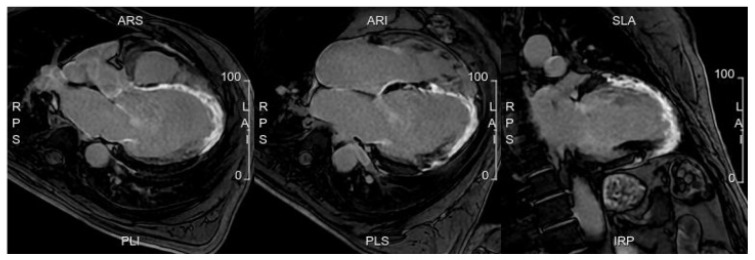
A case from a patient with a recent myocardial infarction. Cardiac MRI evidenced the presence of extensive myocardial left ventricular fibrosis associated with worse prognosis at follow-up.
